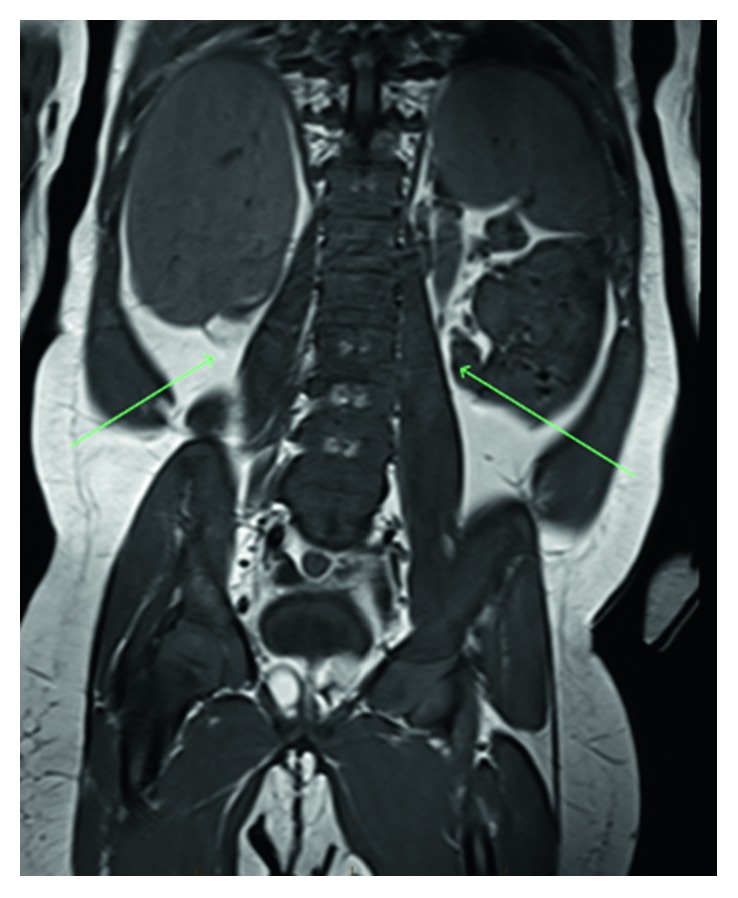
Coronal T1-weighted MRI image showing absence of renal tissue (arrows).
